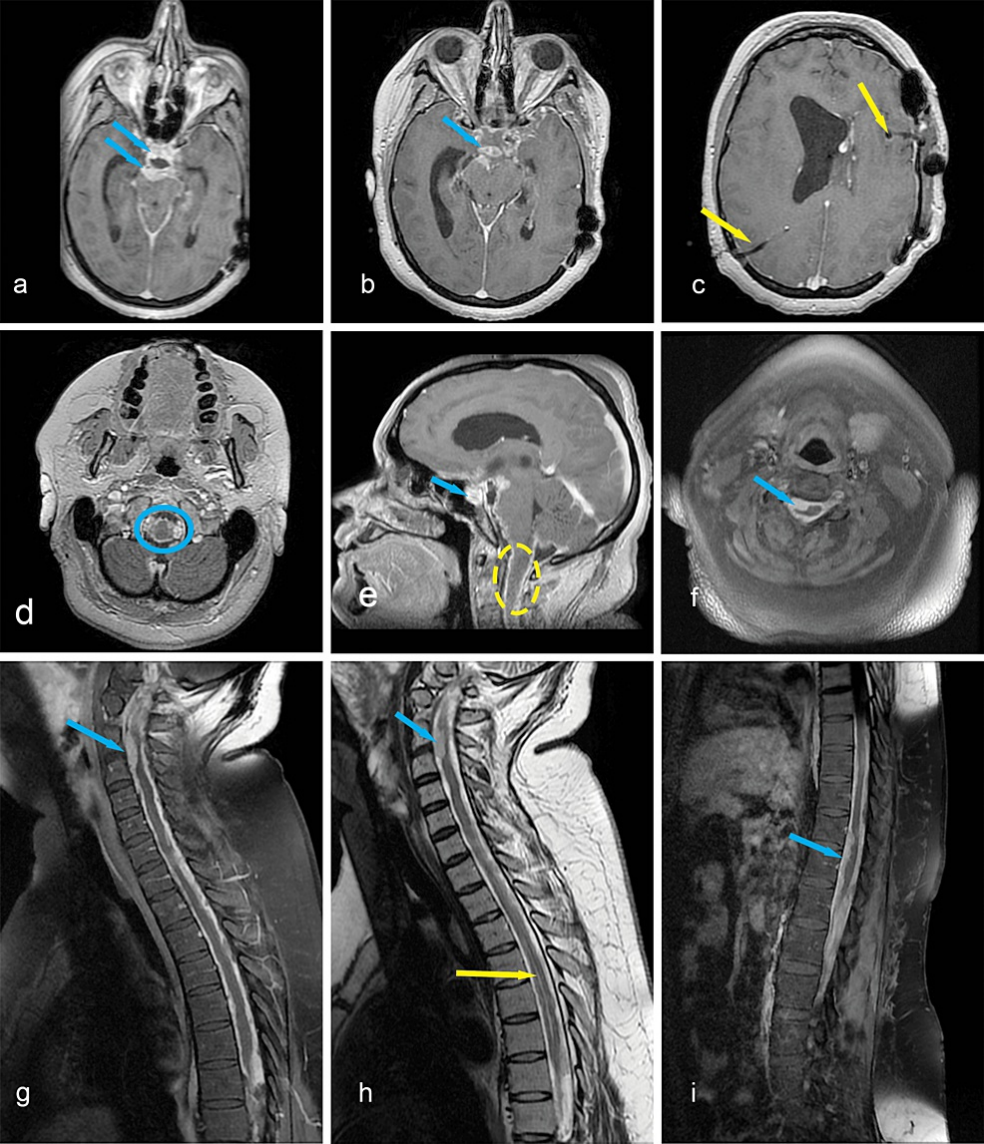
a, b, d: axial contrast-enhanced T1 cerebral MRI depicting leptomeningeal lesions (blue arrows, blue circle); c: axial contrast-enhanced T1 showing the catheters in both lateral ventricles (yellow arrows); e: sagittal contrast-enhanced T1 depicting extensie leptomeningeal enhancement (blue arrow), and the C1/C2 intramedullary lesion persisted, without expansion of the spinal cord, nor contrast enhancement (yellow circle); f: axial contrast-enhanced T1 showing the C3 dural lesion (blue arrow); g, h: contrast-enhanced T1 and non-enhanced T2 cervicothoracic MR image revealing discrete progression, extensive dural thickening (blue arrows), and dilated ependymal canal (yellow arrow); i: thoracolumbar contrast-enhanced T1 image showing extensie dural thickening (blue arrow)
